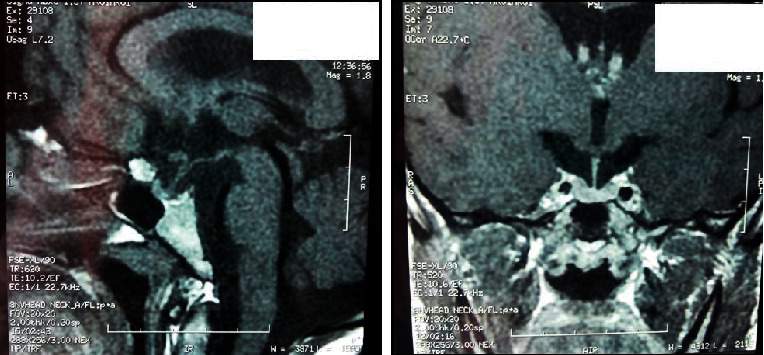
MRI scan showing a small and homogeneous anterior pituitary gland with a partially empty sella turcica.
